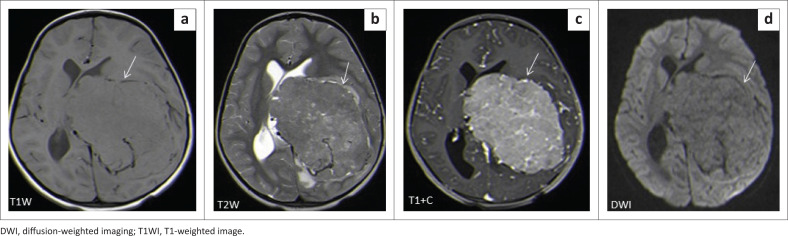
Choroid plexus carcinoma (a–d). There is a large T1 and T2 isointense mass with intense heterogeneous enhancement within the left lateral ventricle causing midline shift to the right. It shows no restriction at DWI.
